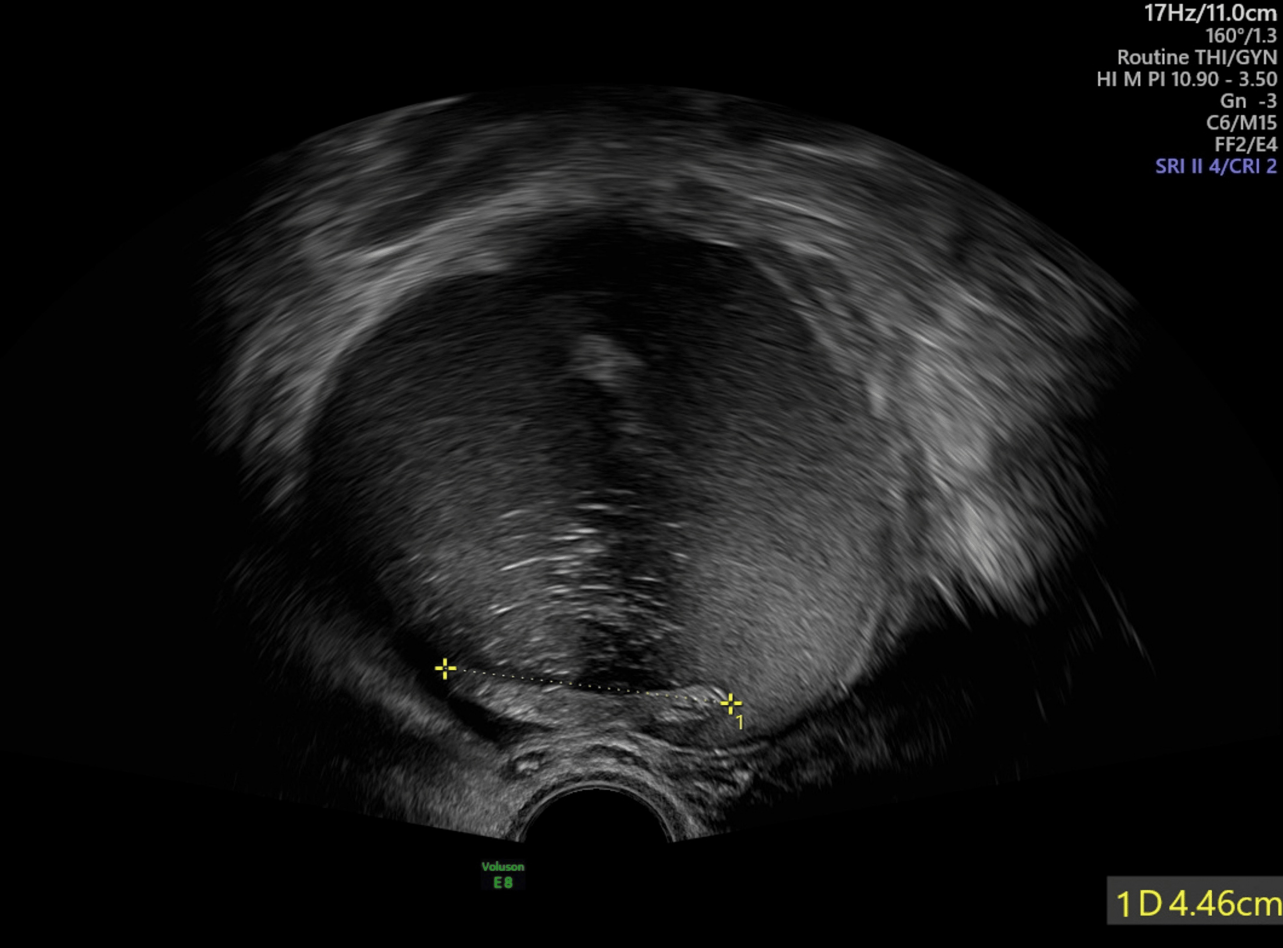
Abdominal ultrasound depicting the mass of 10 cm in diameter
